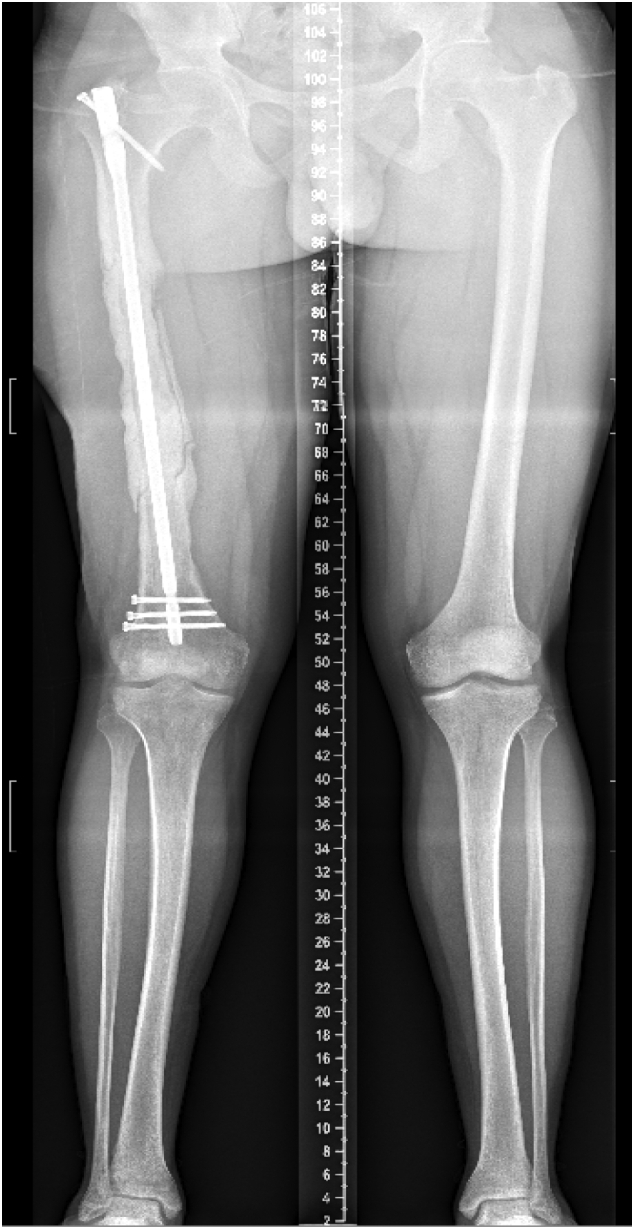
Patient's radiographs upon presentation to our clinic demonstrating a 20 cm segmental defect of his right mid-shaft femur with intramedullary nail and cement spacer in place.
